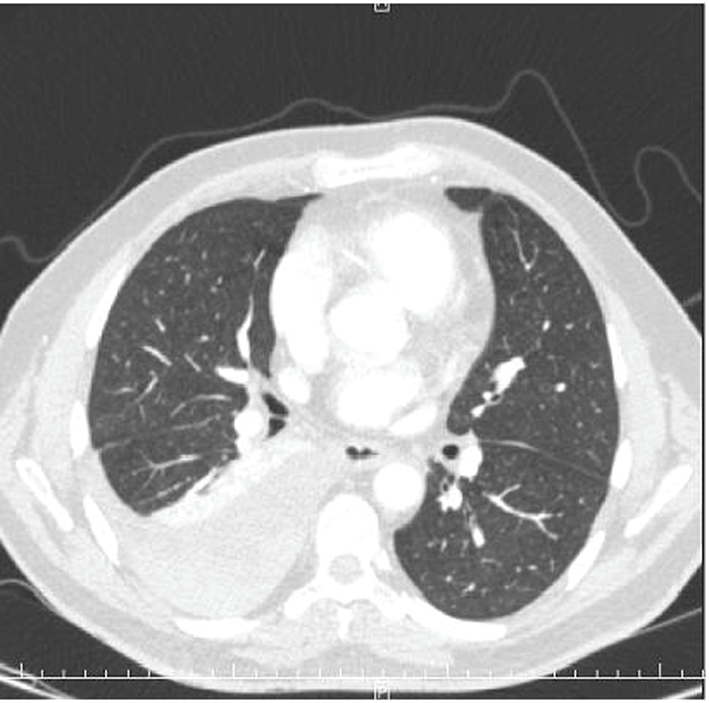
Cervicothoracicangio-CT showing a moderate right pleural effusion with underlying passive atelectasis.
